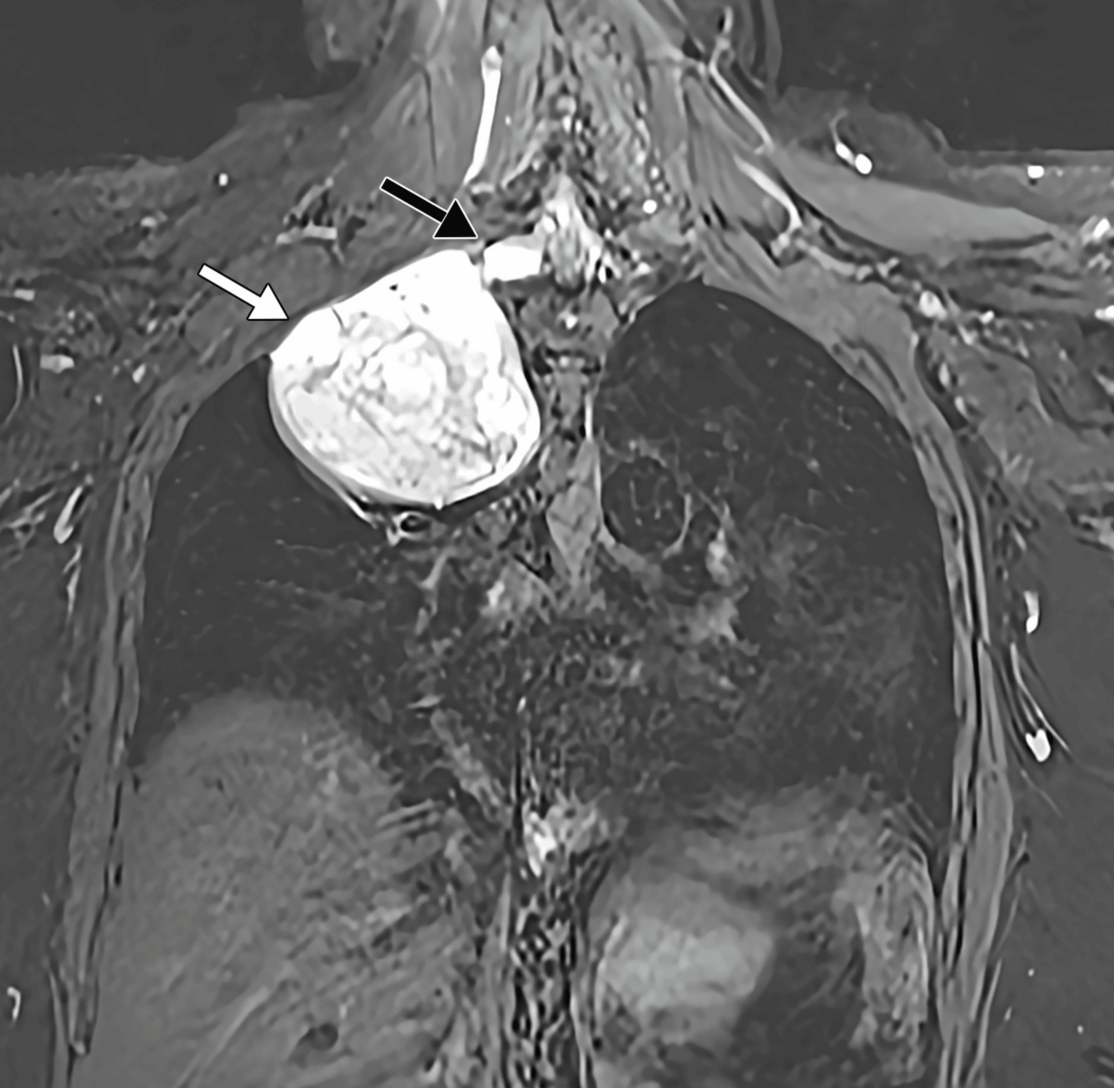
MRI image showing the dumbbell tumour (white arrow) with a smaller spinal portion of the tumour (black arrow). MRI: magnetic resonance imaging.
